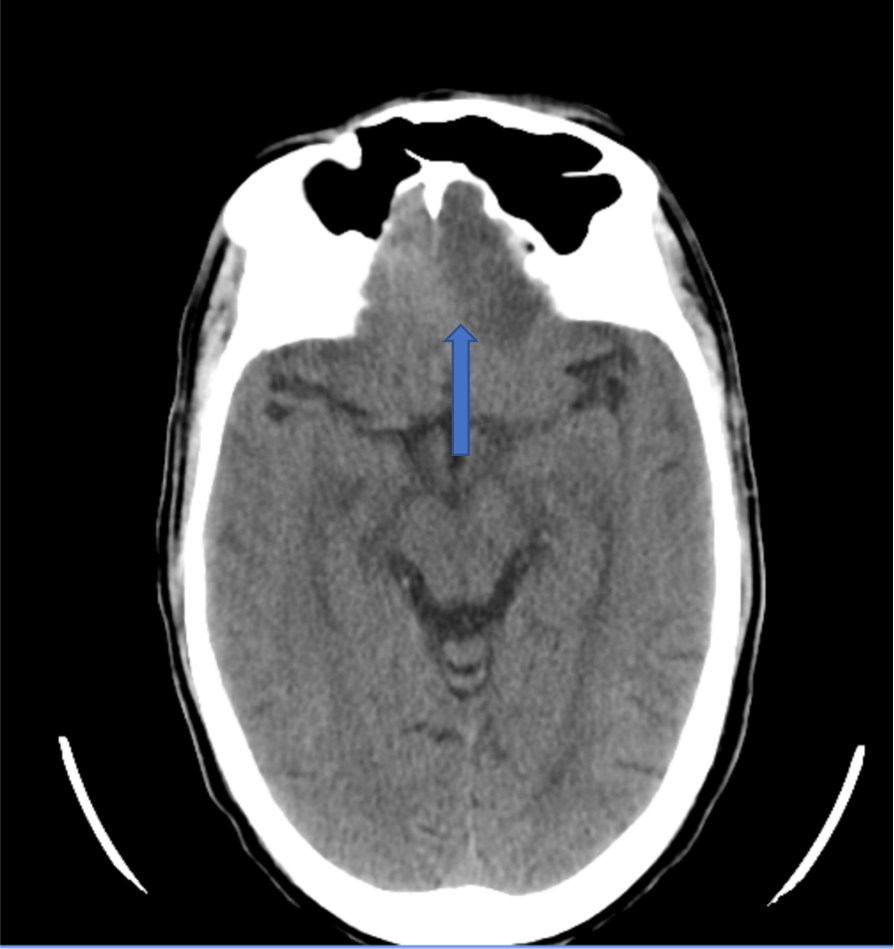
Non-contrast CT scan of the brain revealing a mild midline shift to the right in the frontal region
